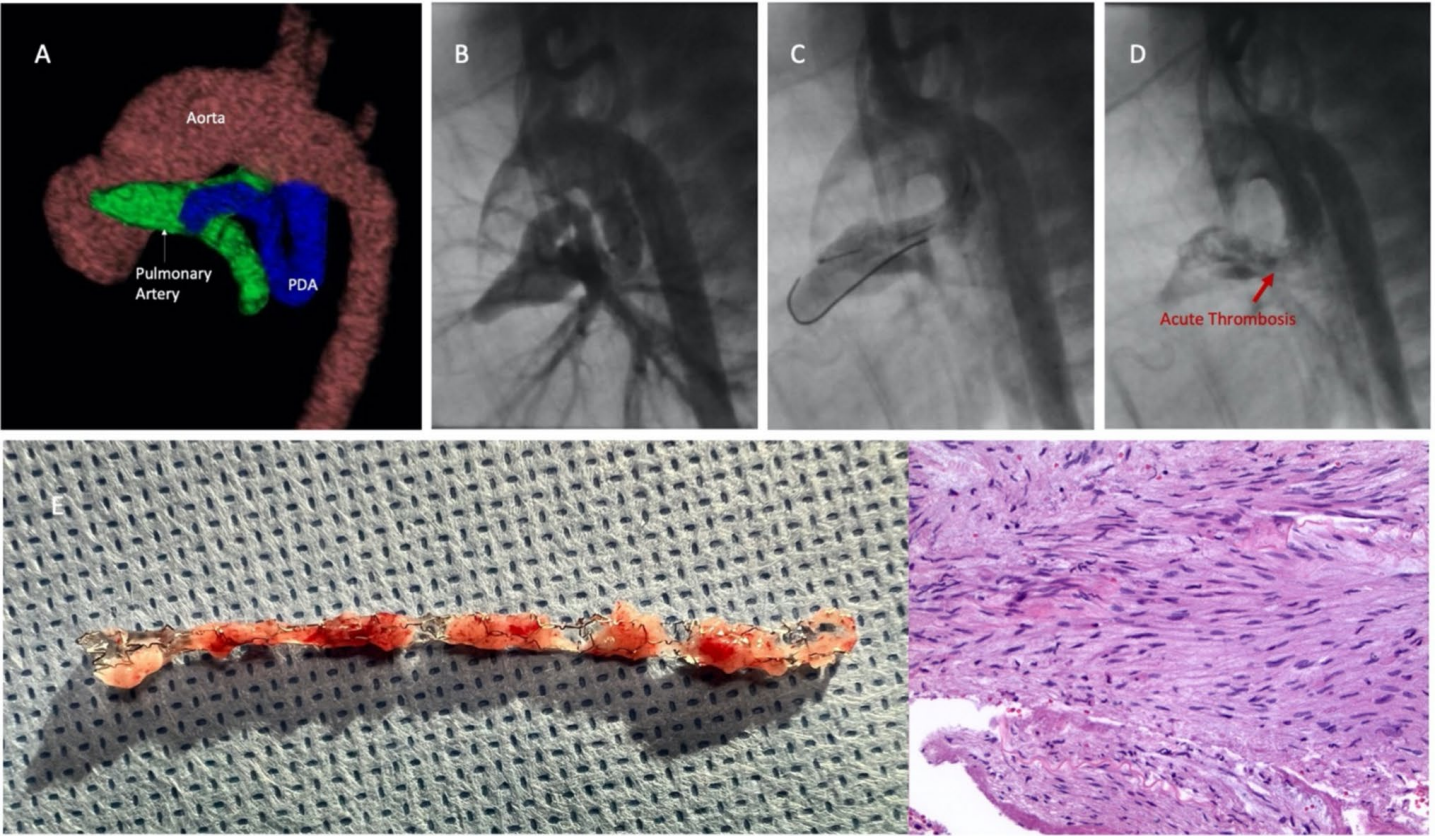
A 3D reconstruction from CT angiogram showing tortuous PDA. B–D Procedural angiograms from lateral projection showing baseline anatomy, patency after initial stent, and acute occlusion after second stent. E Explanted stent with pathologic review showing soft tissue associated with a small portion of vessel wall, blood, and proteinaceous material without evidence of clot organization
